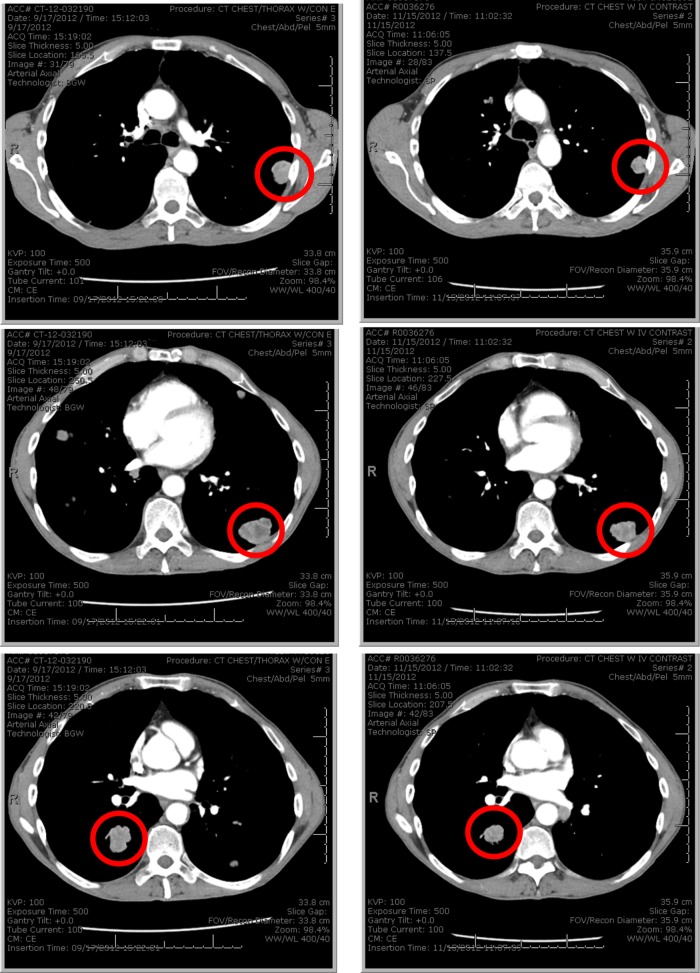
Example of minor radiographic response from ARQ 761. Patient had heavily pre-treated bladder cancer (five prior lines of therapy). Images show multiple pulmonary metastases that decreased in size after initiation of study treatment
